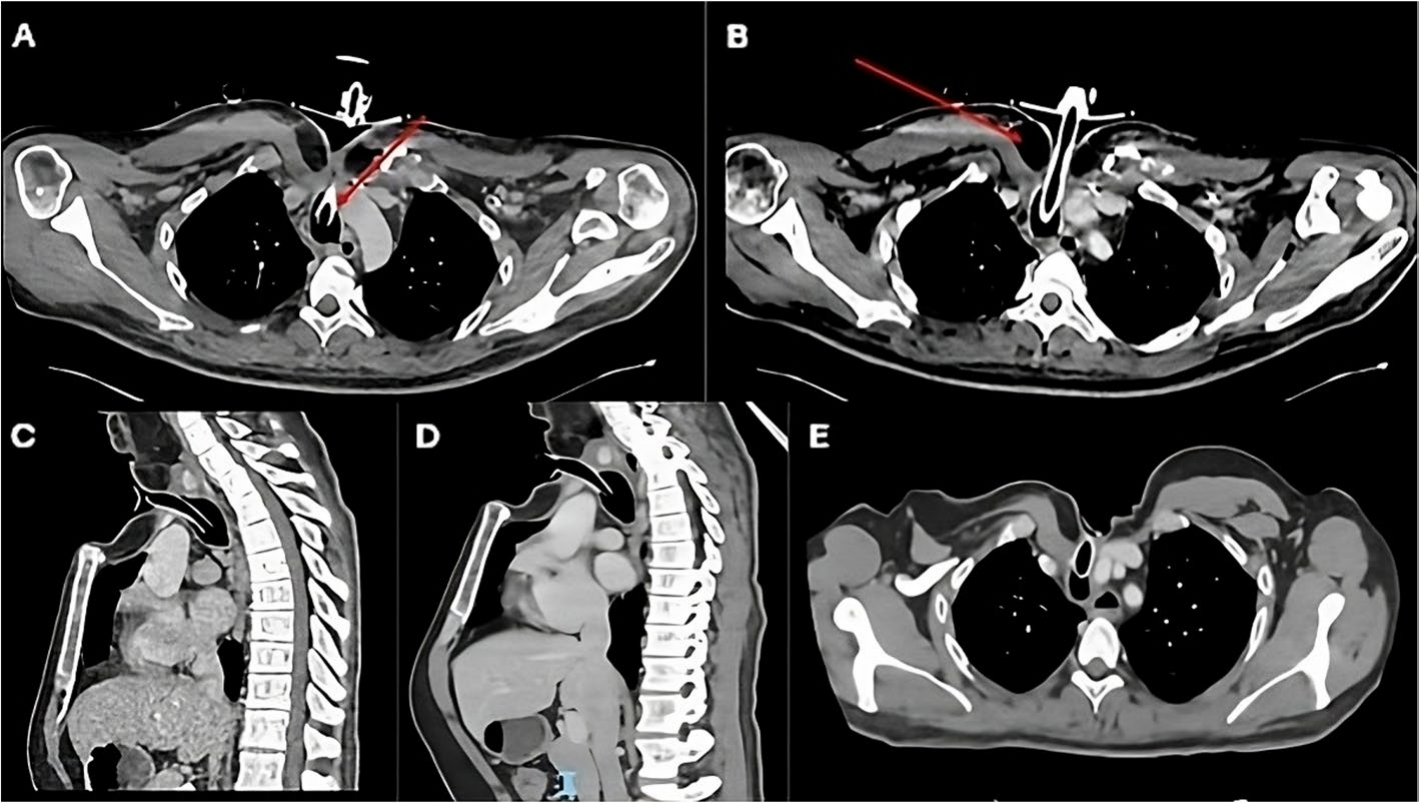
Post-operative CT scan following AMT; A and B demonstrate the mediastinal tracheostomy, through the circular opening in the Pectoralis Major flap (red arrow). Sagittal sections (C and D) demonstrating tracheostomy tube entering distal trachea through the muscle flap. Axial section below level of the tracheostoma (panel E)
